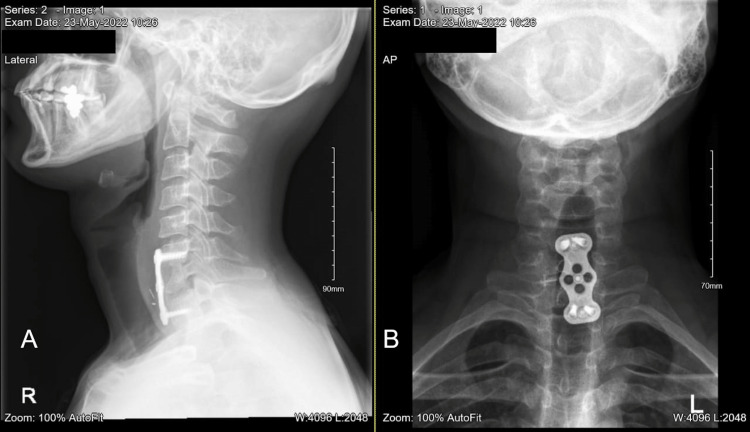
Cervical X-ray four months post-op also shows maintained alignment with signs of union and no signs of hardware complications. (A) Lateral view, (B) AP view.
